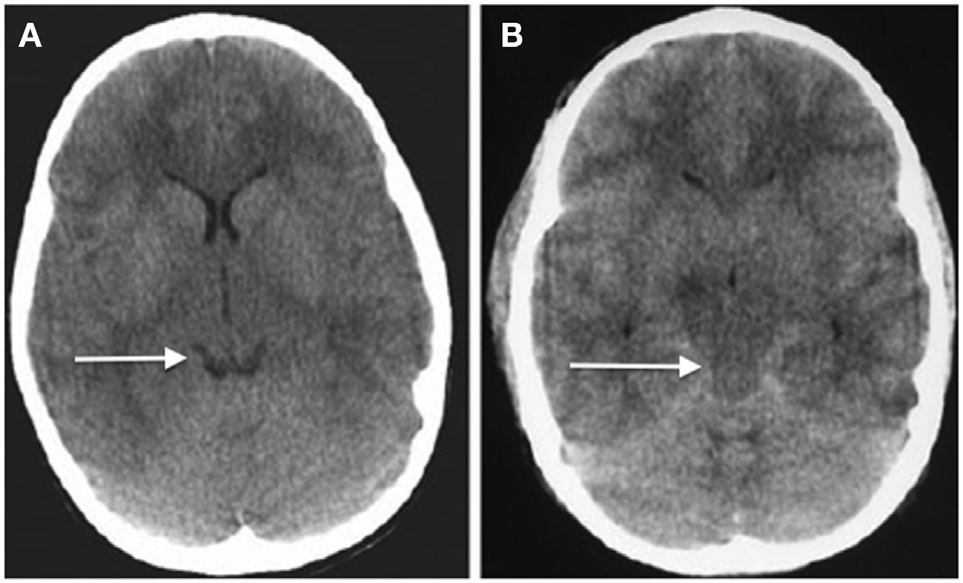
Axial head computed tomography (CT) scans showing (A) relatively normal looking hemispheres with open basal cisterns (arrowed, “smiling brain”) and (B) diffuse severe swelling and obliterations of the cisternal spaces (arrowed) (74) (modified).
